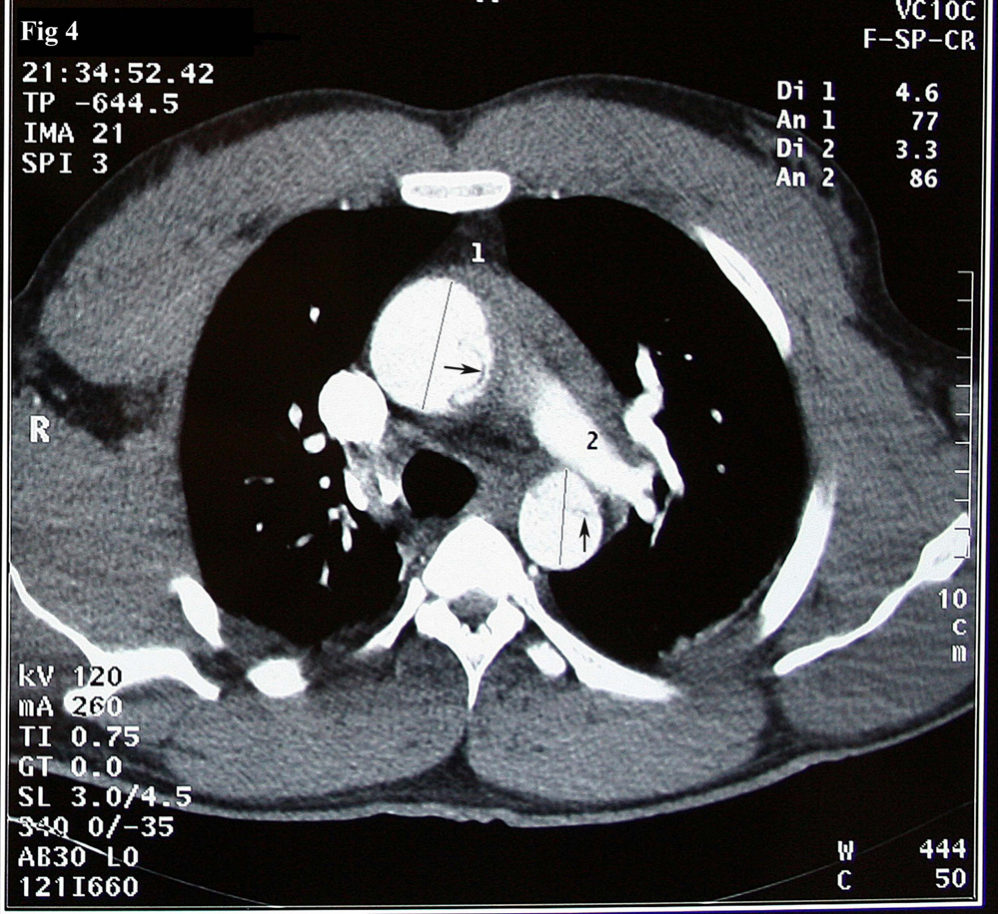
Axial source image of CT angiography show aneurysmal dilation of ascending aorta along with type A aortic dissection.
